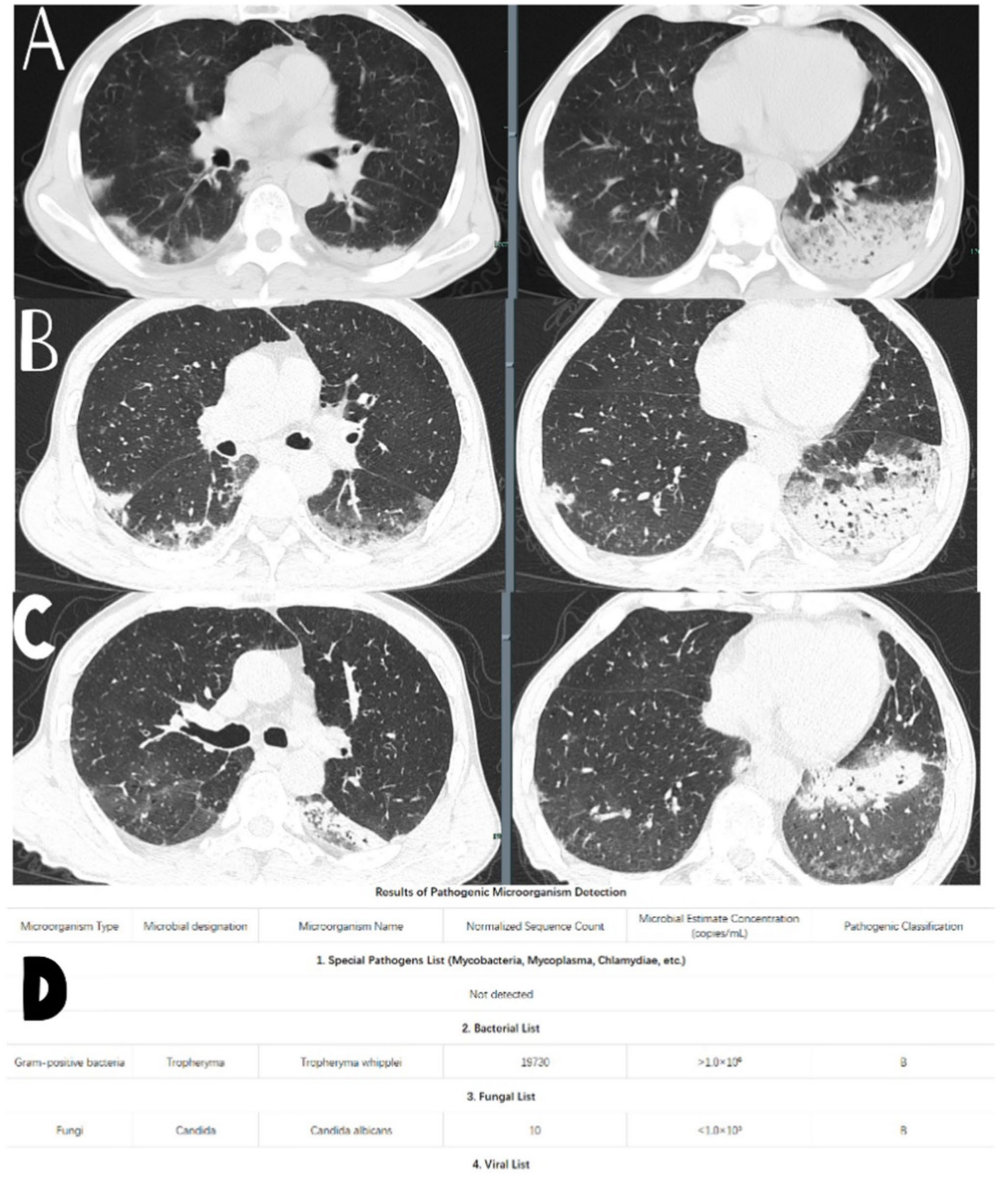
(A) Chest CT performance when the patient was admitted to the hospital. (B) Chest CT performance on the 7th day of admission. (D) Test results of the tNGS. (C) Chest CT performance after 1 month of regular treatment of the patient.
